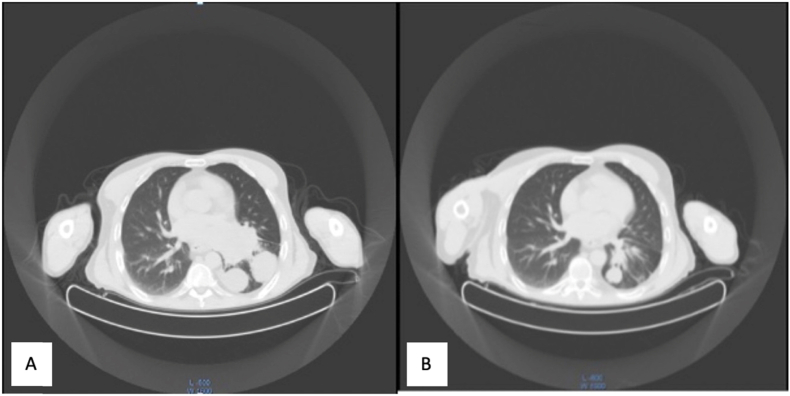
A: CT/PET scan at diagnosis: showed three left lung lesions. B: CT/PET scan 12 months of using keytruda: showed significant regression of these lesions.
